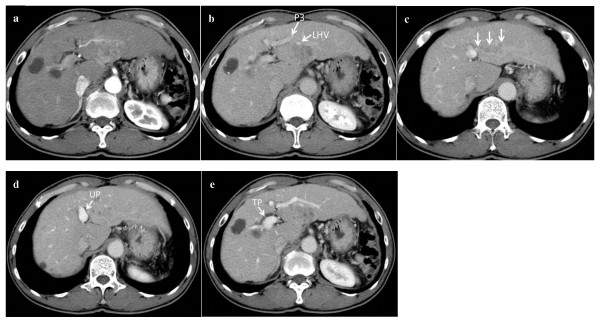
Dynamic computed tomography revealed a tumor in segment 2 of the liver, which was high and low density in the arterial (a) and venous phases (b), respectively, and portal vein tumor thrombus in the portal branch root of segment 2 (arrow) (c). Portal vein tumor thrombus did not invade to umbilical and transverse portions (UP and TP) of the left portal vein (d, e). P3, portal branch of segment 3; LHV, left hepatic vein.
